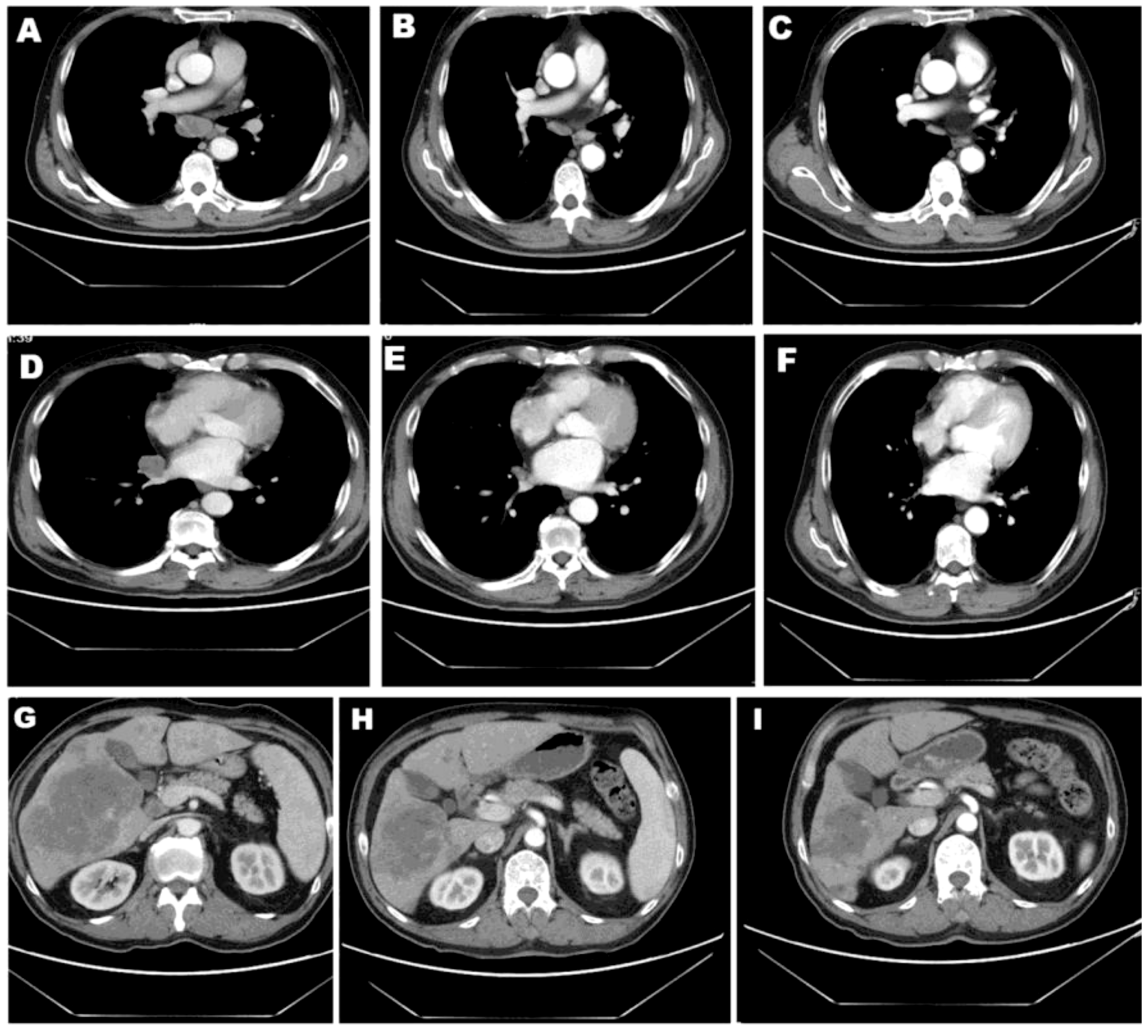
Patient CT images. CT scans of lesion 1 (A–C) and lesion 2 (D–F) in lung as well as lesion in liver (G–I) before and after 1 or 2 months of pralsetinib treatment.
